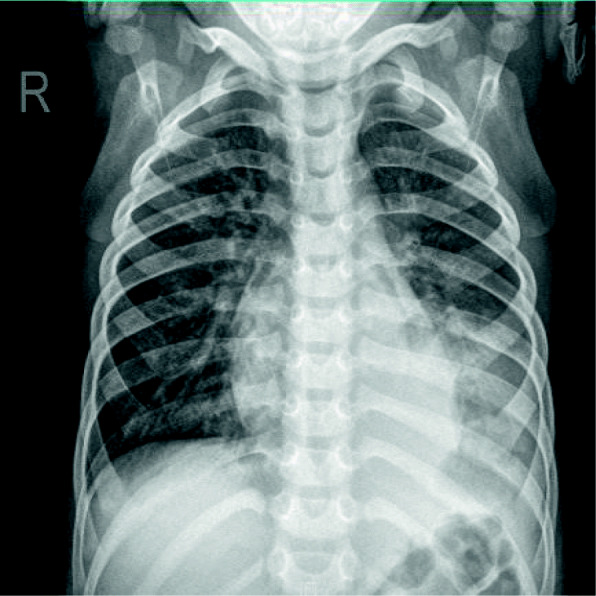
Chest X-ray of patient 2 atelectasis in the left lower lung
